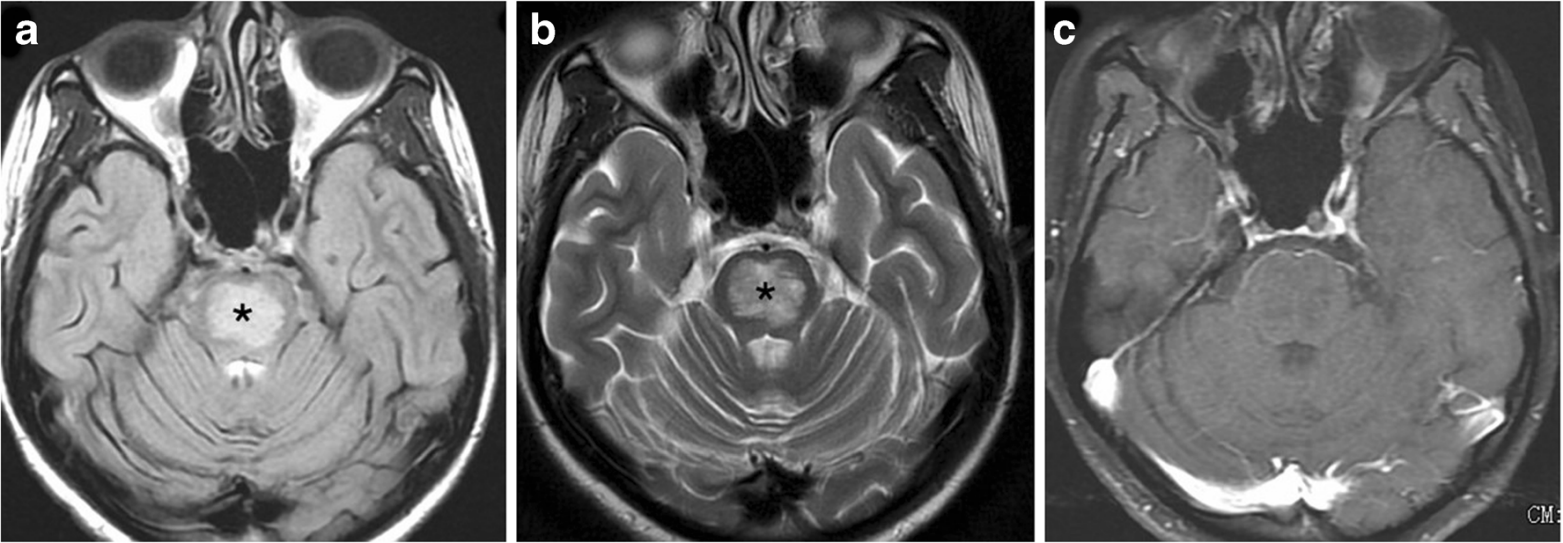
A 15-year-old female with electrolyte imbalance and altered sensorium: a–b Axial FLAIR and T2-weighted images show increased signal in central pons (*asterisk*) with characteristic sparing of the periphery. c No significant post-contrast enhancement is seen
